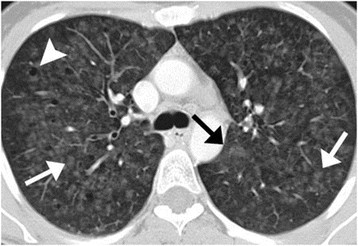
RB-ILD in a 44-year-old woman with a 20 pack-year smoking history. HRCT image of the upper lung lobes shows centrilobular nodules (white arrows), patchy ground-glass opacities (black arrow), and mild coexisting centrilobular emphysema (arrowhead). *Mueller-Mang C, Grosse C, Schmid K, et al.: What every radiologist should know about idiopathic interstitial pneumonias. *Radiographics* 2007, 27:595–615. With permission from The Radiological Society of North America.
